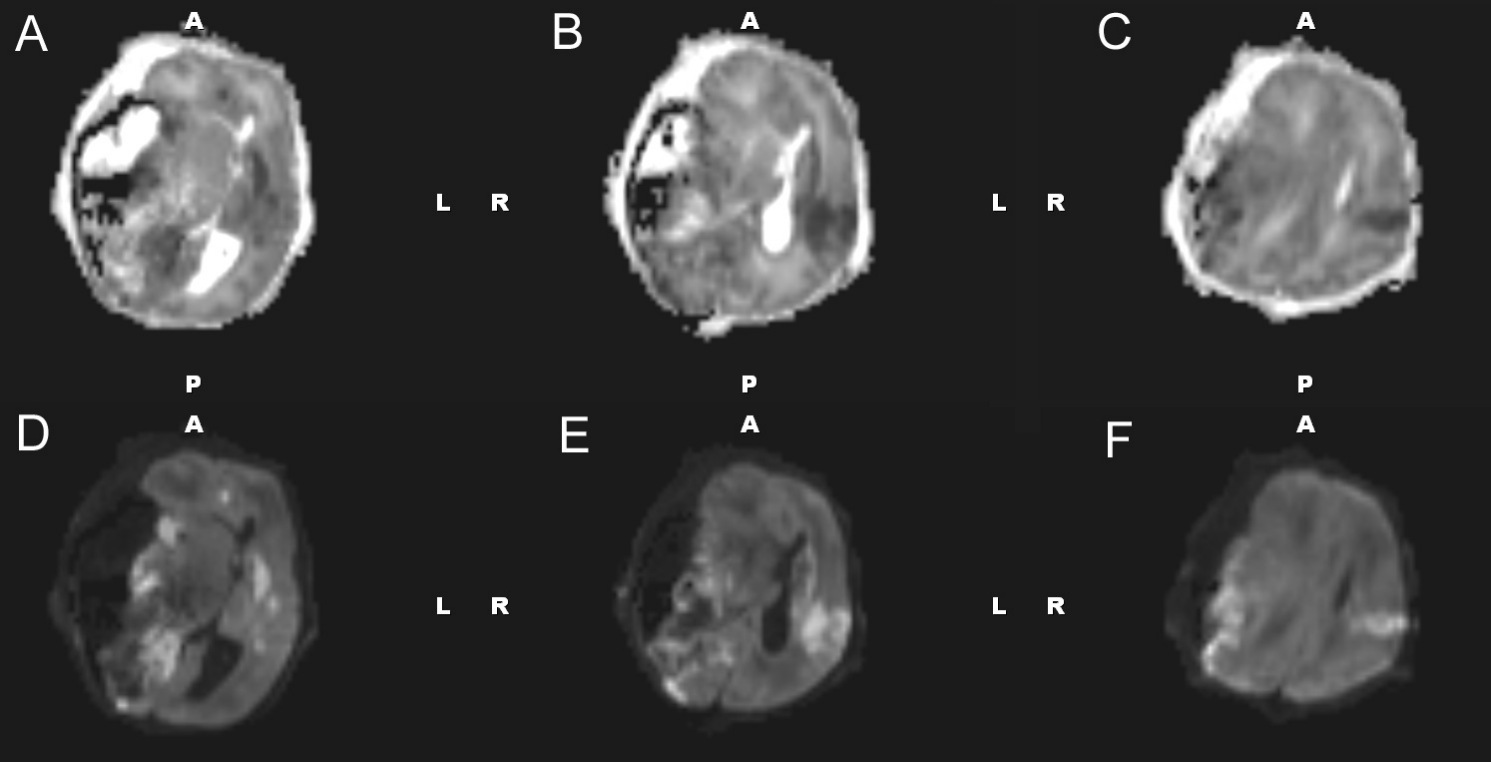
MRI of the brain – Diffusion and apparent diffusion coefficient imaging. Diffusion (A-C) and apparent diffusion coefficient (D-F) axial views characterizing the subdural hematoma.
